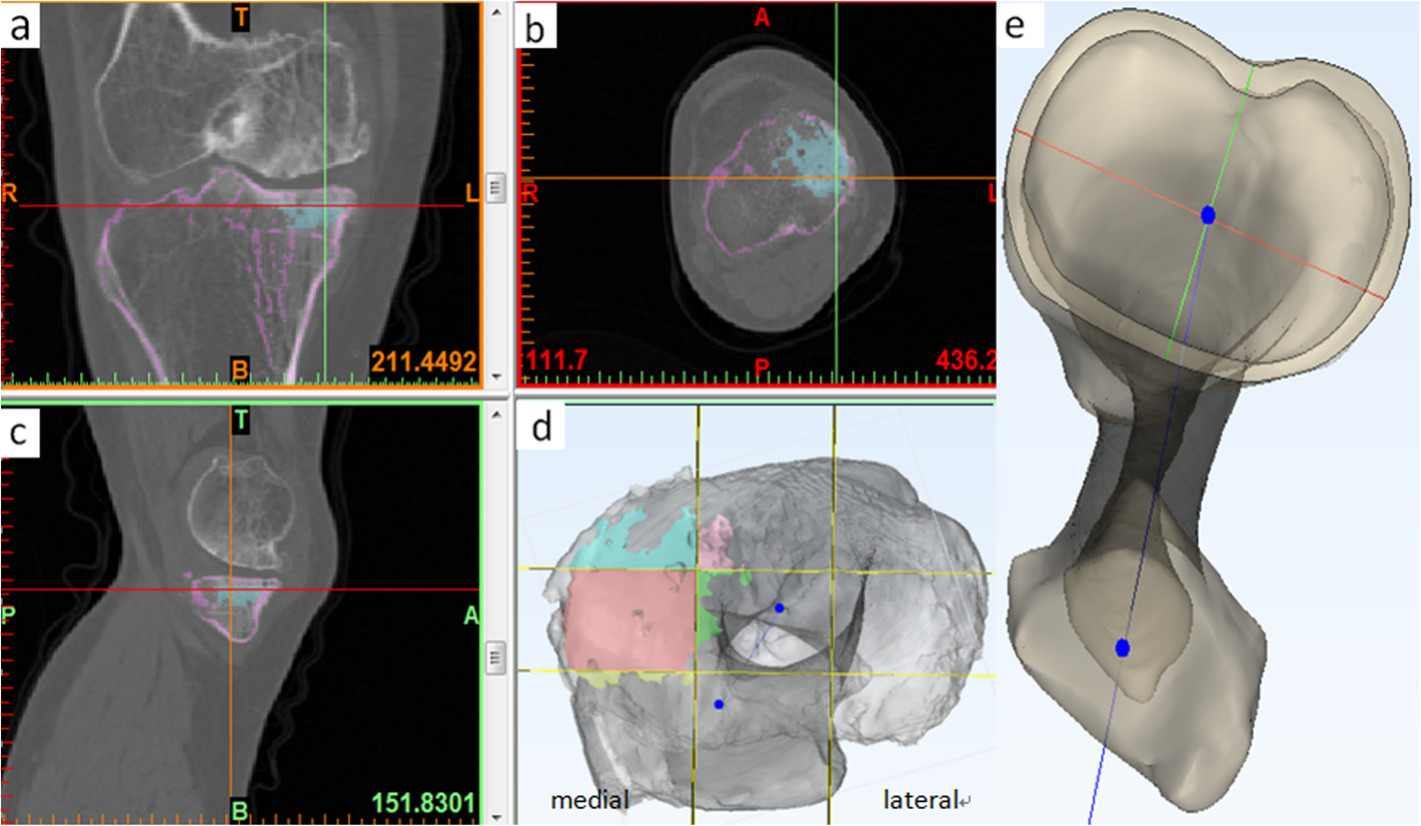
The proximal tibial sclerotic bone area of a severe right varum genu from CT images and the distribution obtained by the 3D reconstruction technique. T: top; B: bottom; L: left (medial); R: right (lateral); A: anterior; P: posterior. Blue line: Z axis (Mechanical alignment,); Green line: Y axis(AP axis); Red line: X axis
